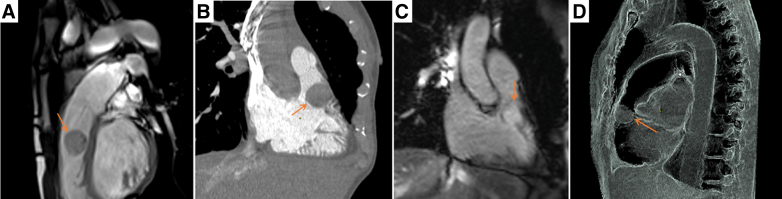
Cardiac imaging. A, Cardiac magnetic resonance imaging (*MRI*) showing a mass in the right ventricular outflow tract below the pulmonary valve (*orange arrow*). B, Computed tomography angiography showing anterior insertion in the pulmonary infundibulum. (*orange arrow*). C, Postgadolinium MRI showing homogeneous enhancement (*orange arrow*). D, Three-dimensional reconstruction of the mass (*orange arrow*).
